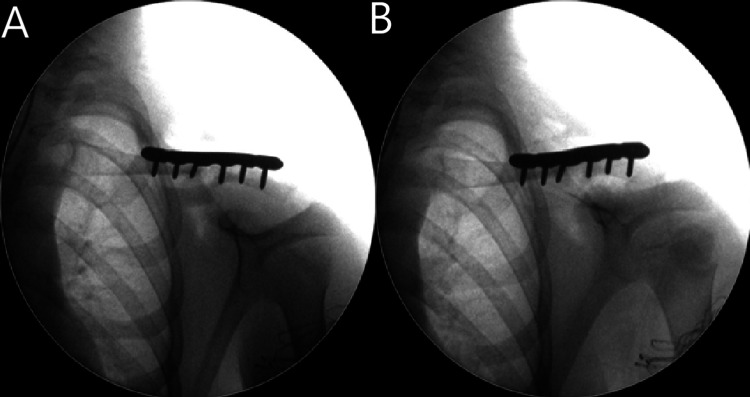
(A) An anteroposterior and (B) oblique, intraoperative radiographs showing clavicle fixation (Case 2)
